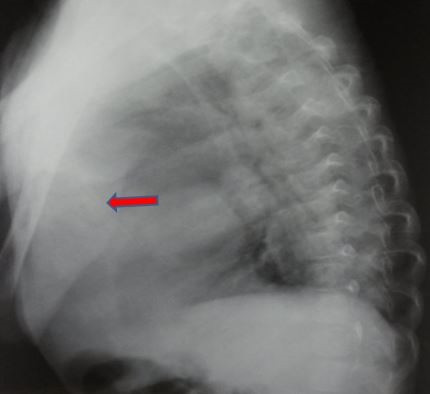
radiographie de thorax profil: confirme le siège antérieur de l’opacité (flèche rouge)
